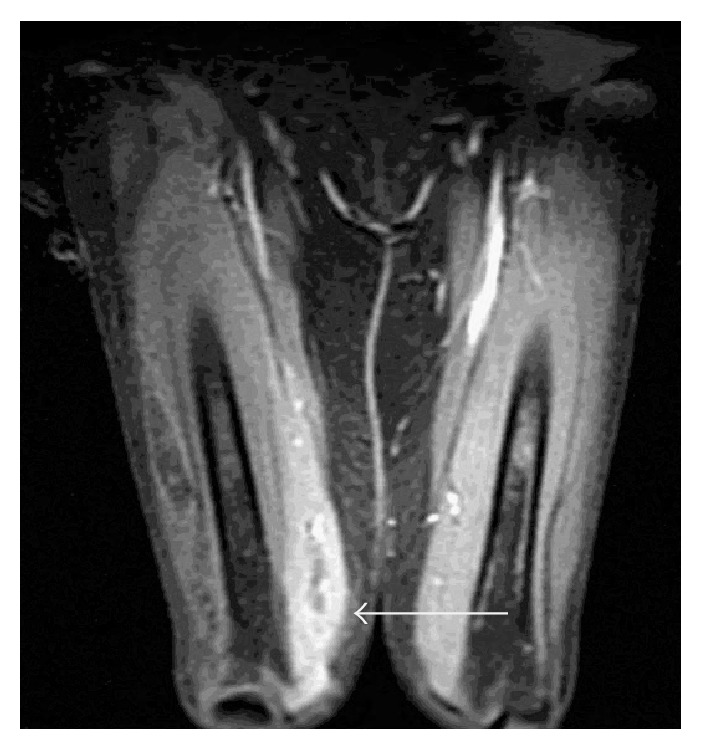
Coronal T2-weighted FAT SAT showing diffuse muscle edema involving the lower two-thirds of the anterior compartment muscles. The left thigh also shows muscle edema especially along the superior aspect of the rectus femoris and inferior aspect of the posterior compartment muscles.
